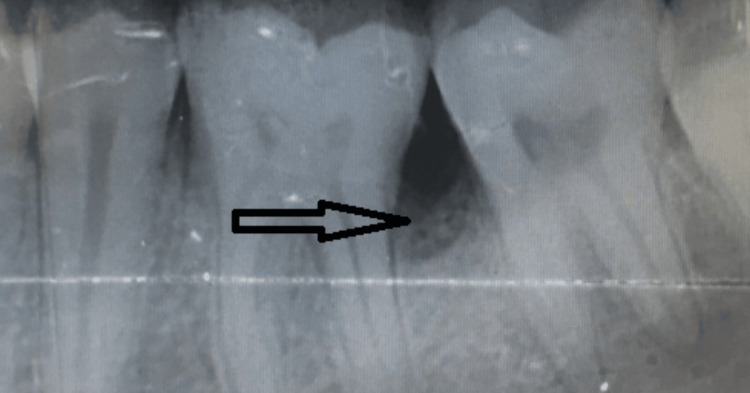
Preoperative radiograph of case 1 The arrow indicates saucer-shaped defect in relation to #36-37
